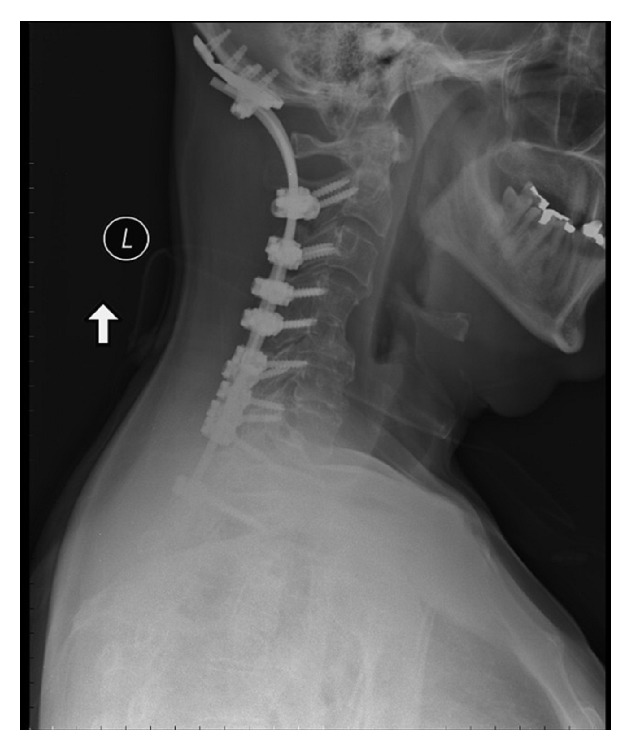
Postoperative lateral X-ray demonstrating posterior instrumentation from the occiput to T2.
